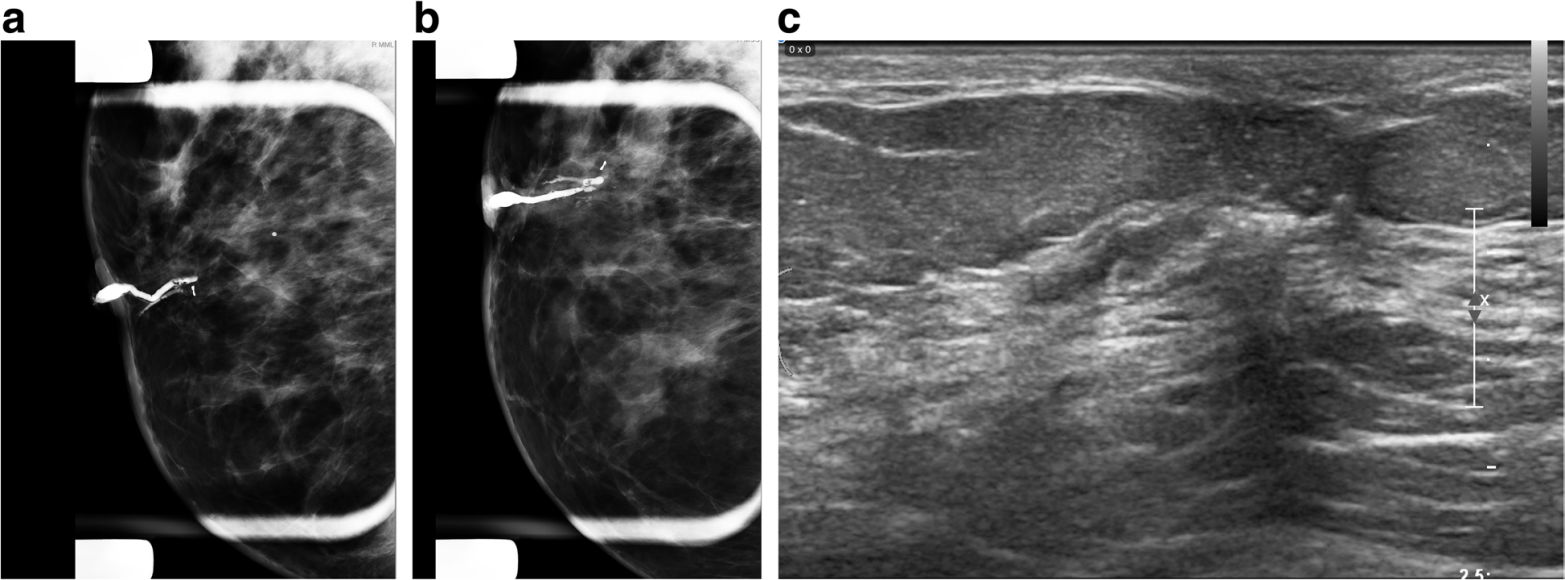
A 50-year-old female presented for evaluation of clear right nipple discharge. Ductography radiographs show an irregular filling defect in the subareolar duct. The post-biopsy marker clip from a previous biopsy was demonstrated adjacent to the site of the filling defect. The patient was returned to the US room. The selected duct within the right breast was distended with contrast and better seen. It appeared to terminate at an isoechoic intraductal mass at the 6:00 subareolar position. A US-guided biopsy of this lesion was performed. The pathology result showed benign papilloma with mild usual ductal hyperplasia and no evidence of atypia
